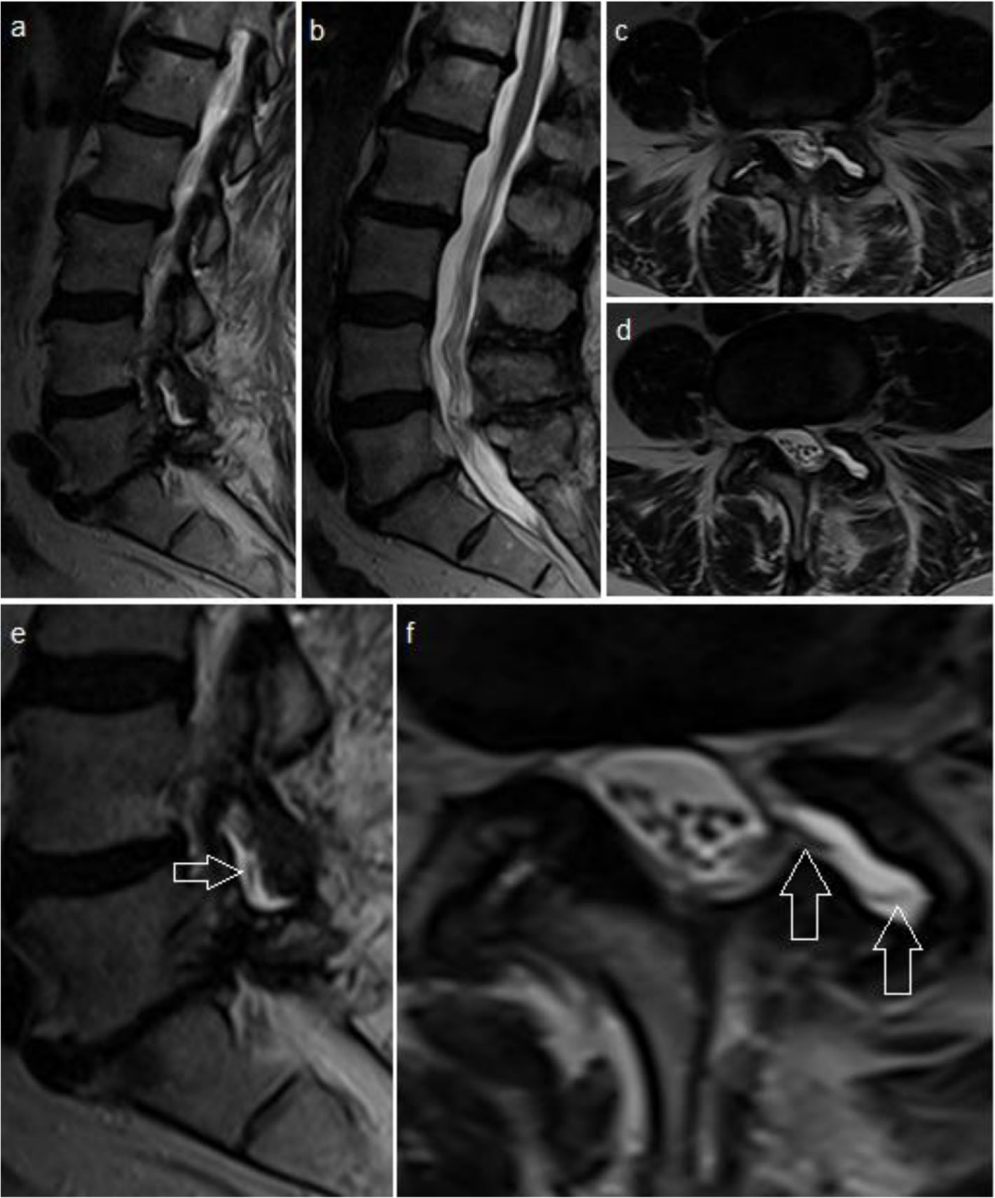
MRI after decompression surgery and sudden sciatica onset: Sagittal (a-b) and axial (c-d) T2 weighted images showing no hematoma or intraspinal compression. An effusion of the facet joint is noted. Zoomed sagittal (e) and axial (f) image shows herniation of a nerve root (white arrows) into the facet joint gap
